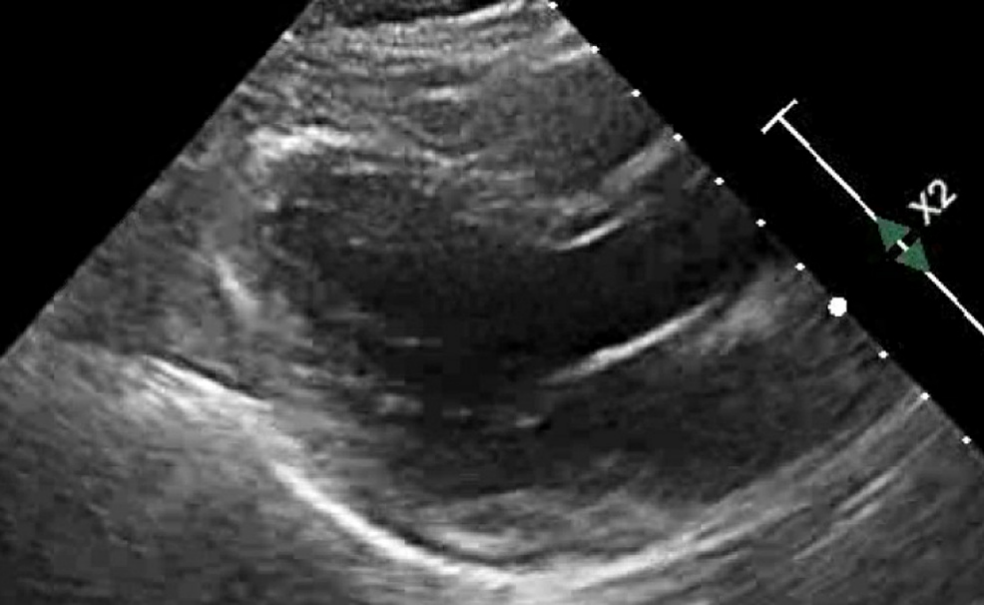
Echocardiogram showing mild pericardial effusion.
